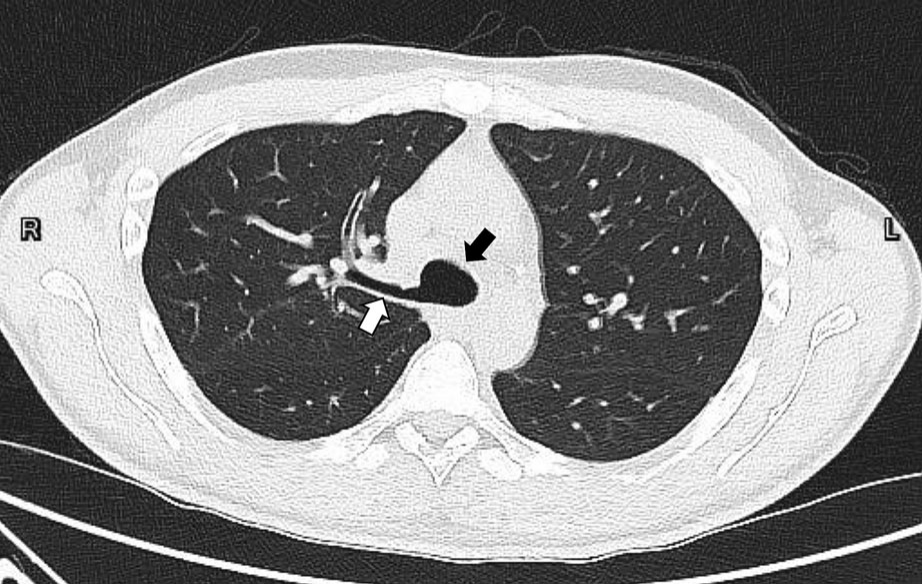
Axial view of chest computed tomography with lung window revealing tracheal bronchus (white arrow) over the right‐sided distal tracheal (black arrow) above the carina with absence of right upper lobe bronchus.
